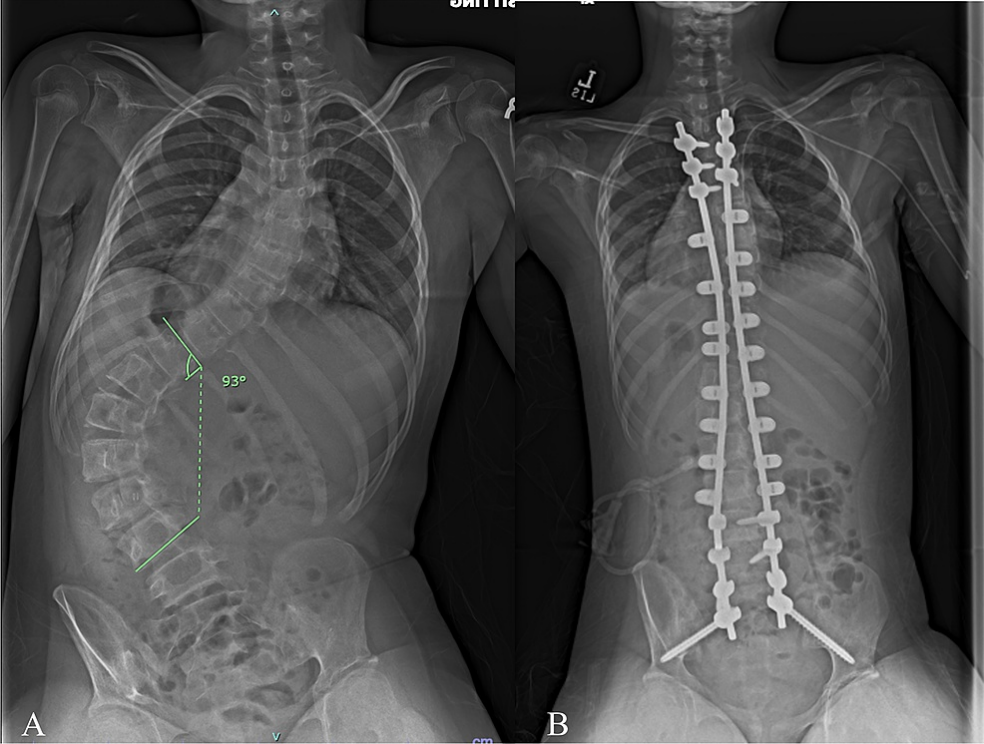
A) Preoperative and B) postoperative sitting PA views. The preoperative x-ray demonstrates a 93 deg left-sided thoracolumbar curve with 11 degrees of pelvic obliquity. The postoperative x-ray demonstrated T3 to pelvis instrumented fusion with the utilization of multiple sublaminar bands. The thoracolumbar curve improved to 9 degrees.
